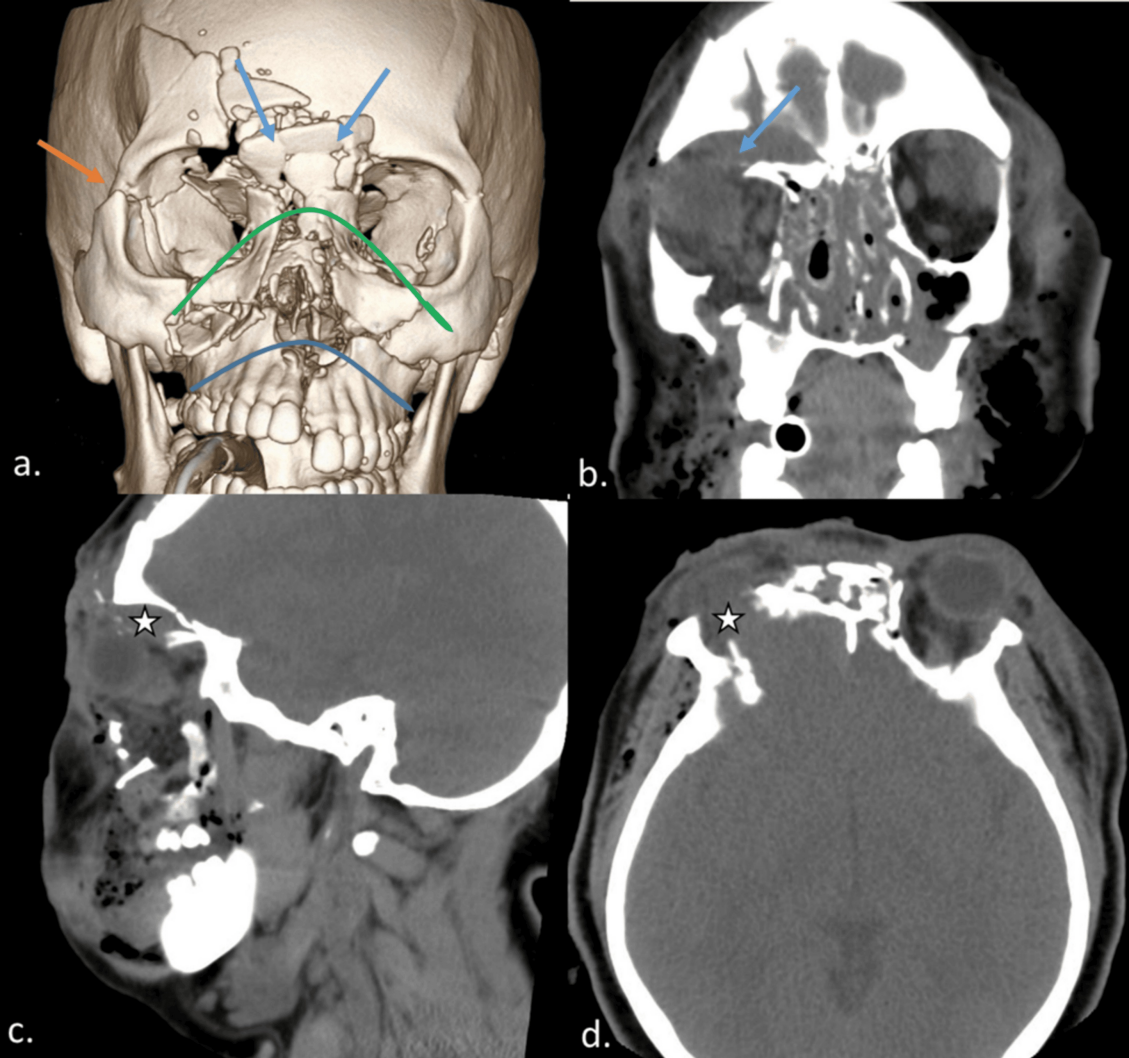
Initial CT at presentation The 3D volume-rendered image (a) demonstrates displaced fractures of the inferior maxillary sinus walls and nasal cavity walls following a LeFort I pattern (curved blue line), as well as displaced fractures of the bilateral medial orbital walls and orbital floors following a LeFort II pattern (curved green line). The right lateral orbital wall is also fractured (orange arrow). Along the central aspect, there are NOE fractures (blue arrows). On coronal reformatted CT soft tissue window images (b), a blow-in fracture of the right orbital roof with a wide fracture defect is apparent (blue arrow). Sagittal plane (c) and axial plane (d) images demonstrate the bulging of the dura (stars) into the superior right orbit through the fracture defect. CT: computed tomography, NOE: naso-orbital-ethmoid
